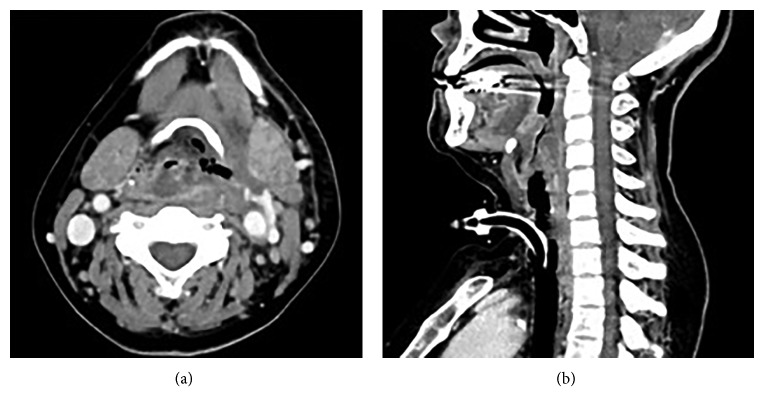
Enhanced CT findings (after tracheostomy). Enhanced CT scan revealed a large mass involving the left parapharyngeal and retropharyngeal space, causing significant airway narrowing.
